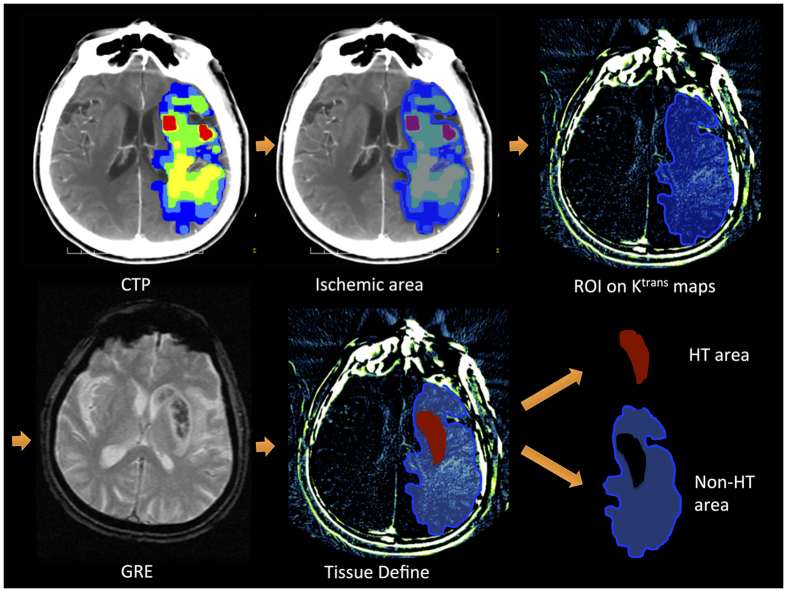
The protocol used to define the regions of interest (ROIs) for both hemorrhagic transformation (HT) and Non-HT areas on K^trans^ maps. Step 1: The perfusion computed tomography (CTP) output images were used to draw the distribution of the ischemic area manually. Step 2: The ischemic area was overlapped on the K^trans^ map to determine the ROIs, and all permeability values were restricted to this area. Step 3: ROIs were divided into HT areas and Non-HT areas, corresponding to the HT regions on the gradient recalled echo (GRE) T2-weighted magnetic resonance image.
